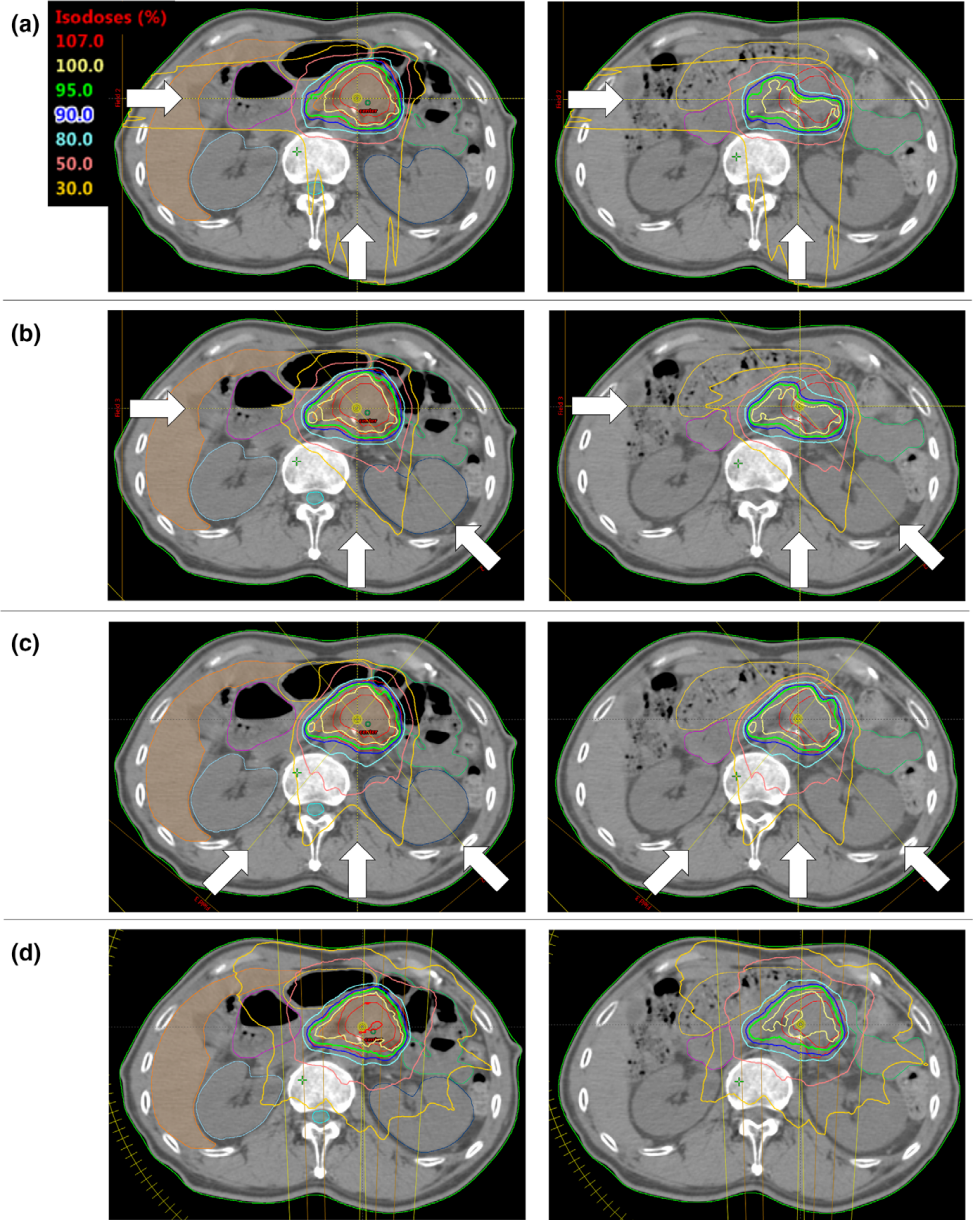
Change in dose distribution. Comparison of the dose distribution between the original plan (left) and the plan assigned to the first additional CT (right) in (a) RP, (b) RPOL, (c) ORPOL and (d) VMAT plans.
